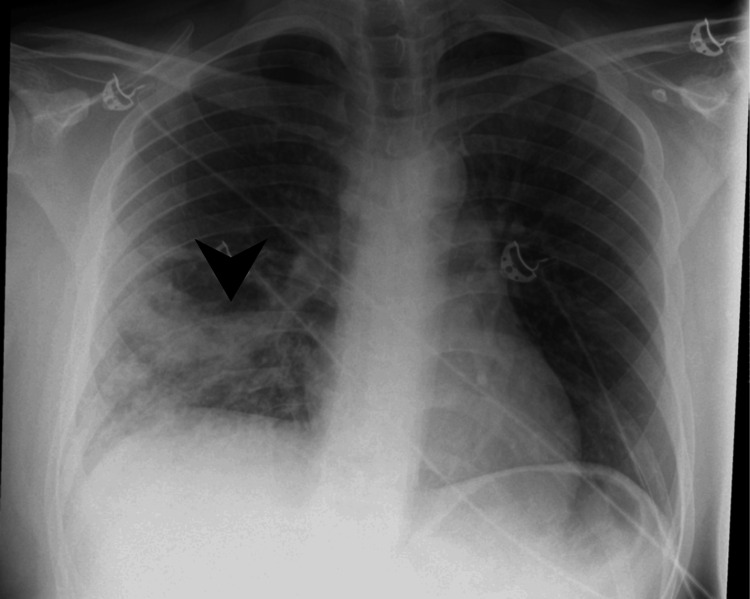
Follow-up posteroanterior chest radiograph showing progression of right lower lobe consolidation (arrowhead). In comparison to the previous image, there is an increase in the extent and density of the right lower lung opacity.
